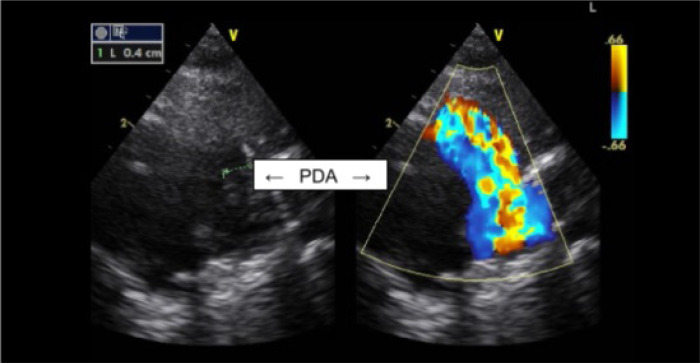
Transthoracic echocardiogram. PDA=patent ductus arteriosus.
